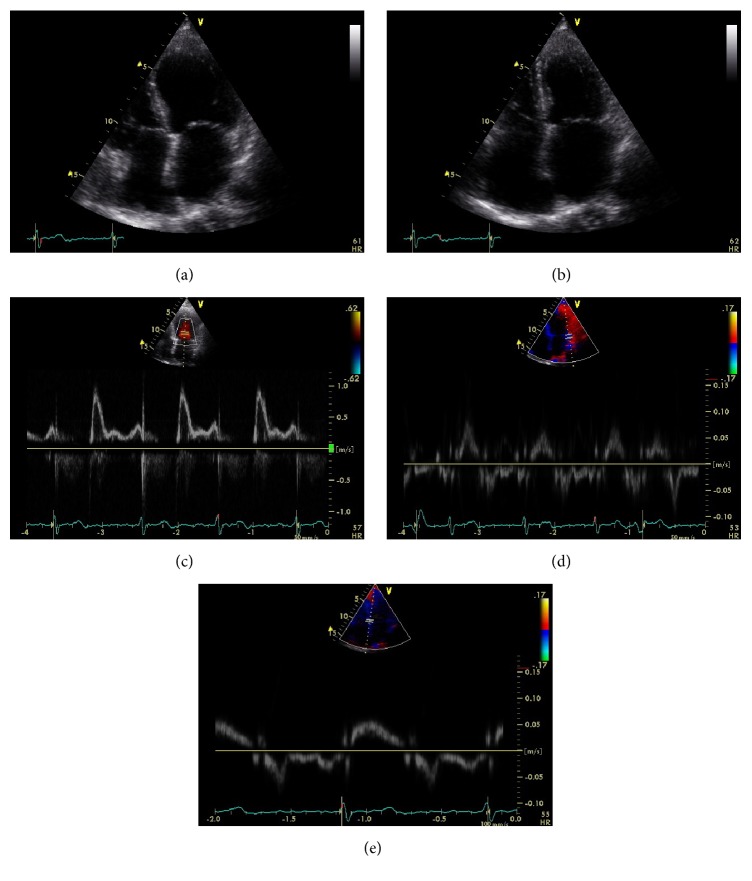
Echocardiographic images from a patient with restrictive cardiomyopathy. Two-dimensional 4-chamber view in diastole (a) and systole (b), showing normal left ventricular volume, wall thickness, and systolic function (EF 62%). There is marked biatrial enlargement. Pulse wave Doppler from the left ventricular inflow (c) showing restrictive filling pattern with an E wave velocity of 1 m/sec, an A wave velocity of 0.4 m/sec, and an E wave deceleration time of 145 msec. Spectral tissue Doppler from the lateral (d) and septal (e) mitral annulus. There is marked reduction in systolic annular velocities indicative of latent systolic dysfunction.* E*/*e*′ (*e*′ measured as the average between the two annular* e*′ velocities) is 16, indicative of increased left ventricular filling pressure. Note the marked reduction in lateral* a*′ velocity (<4 cm/sec) indicative of left atrial systolic dysfunction.
